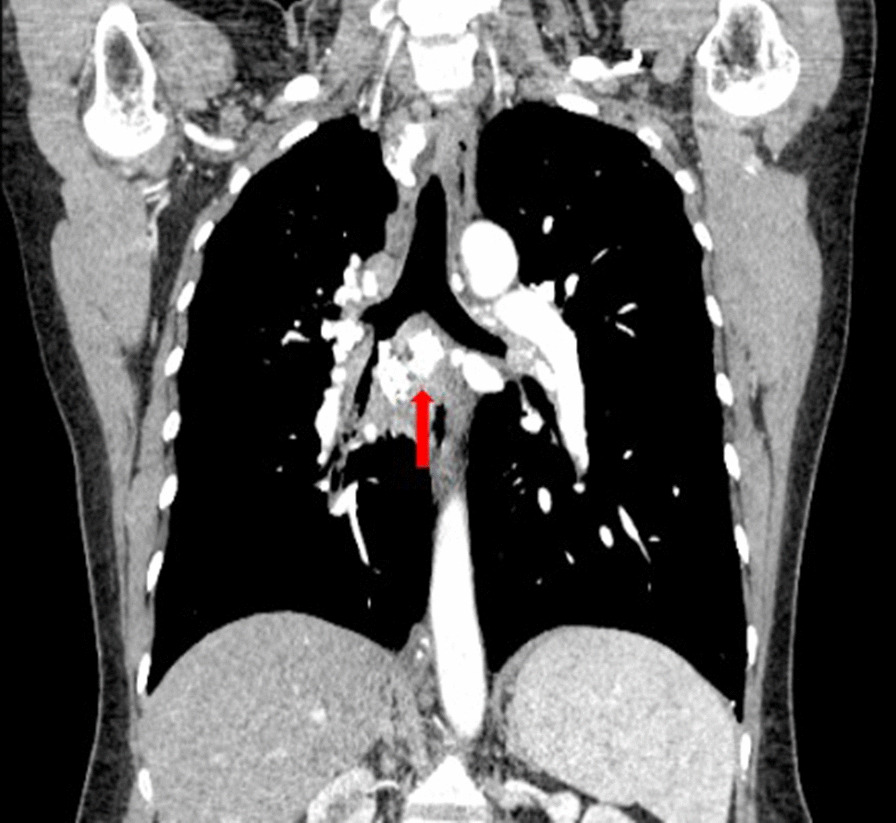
Chest CT scans with contrast show large transbronchial broncholith in the right intermediate bronchus (red arrow) (patient 11, before procedure)
